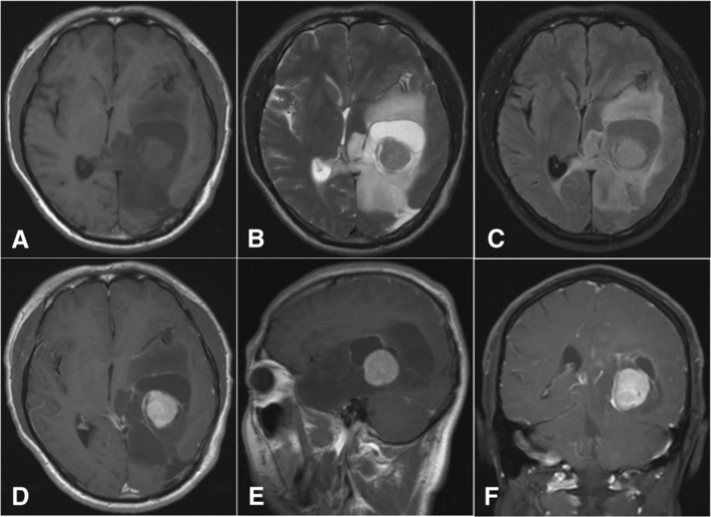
Magnetic resonance imaging (MRI) images recorded in October 2010. Head MRI scan (A-C) and enhanced MRI (D-F) images showing the enhancement on the cystic tumor wall and the lesion in the center of the cystic tumor.
